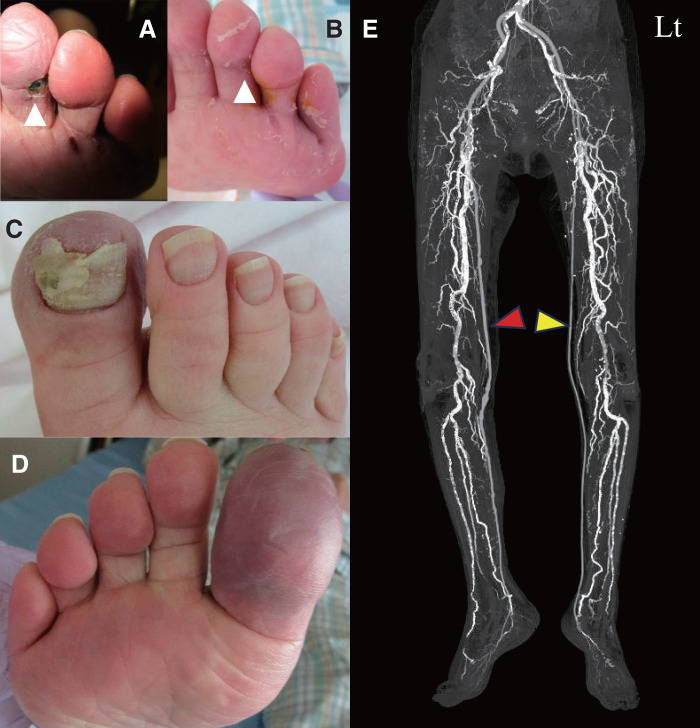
Bilateral foot before bypass surgery and CTA after bilateral bypass surgery. Left foot ischemic ulcer (A). Healed left foot ischemic ulcer after bypass surgery (B). Right cyanotic dorsal foot (C). Right cyanotic plantar foot (D). CTA images obtained following bypass surgery showing patent bilateral bypass grafts (red arrowhead, right; yellow arrowhead, left; E). CTA: computed tomography angiography; Lt: left
